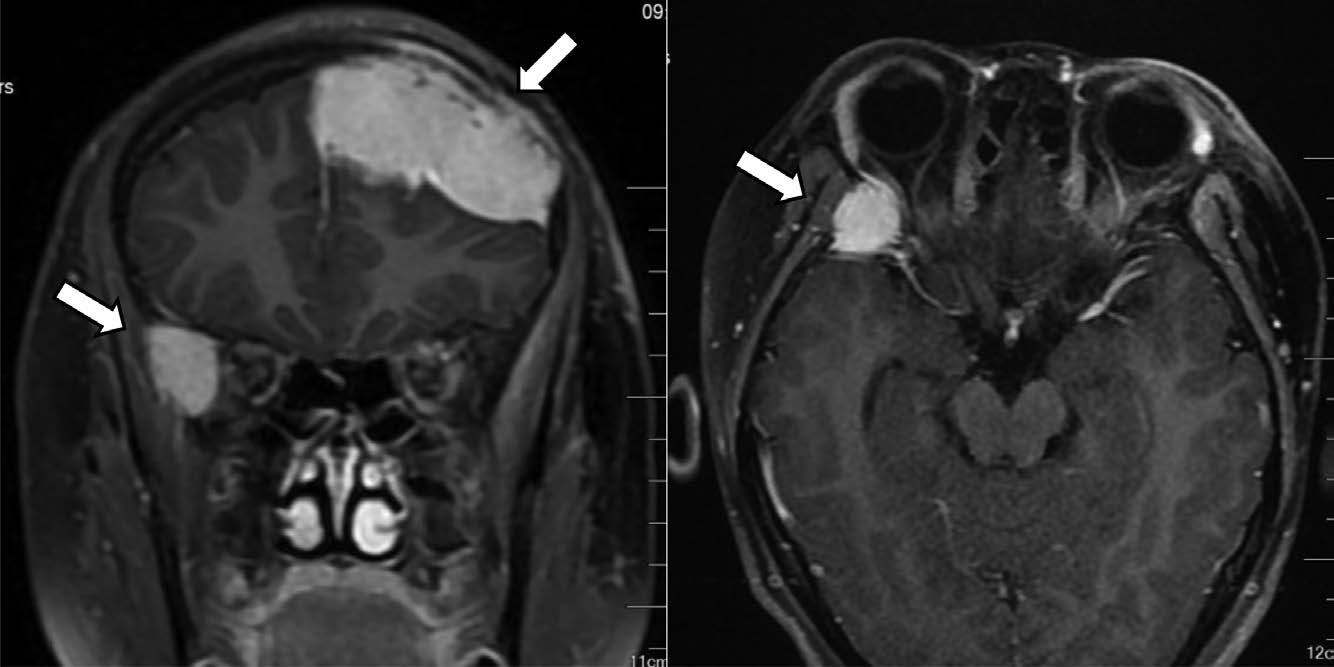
MRI showed that the enhancement focus of the left frontal bone was larger than before, with a cross section of 3.7 cm*3.1 cm. Nodular long T1 and long T2 abnormal signals were found in the right temporal region, ranging from 1.6 cm*2.2 cm, and the enhanced scan showed obvious enhancement. MRI = magnetic resonance imaging.
